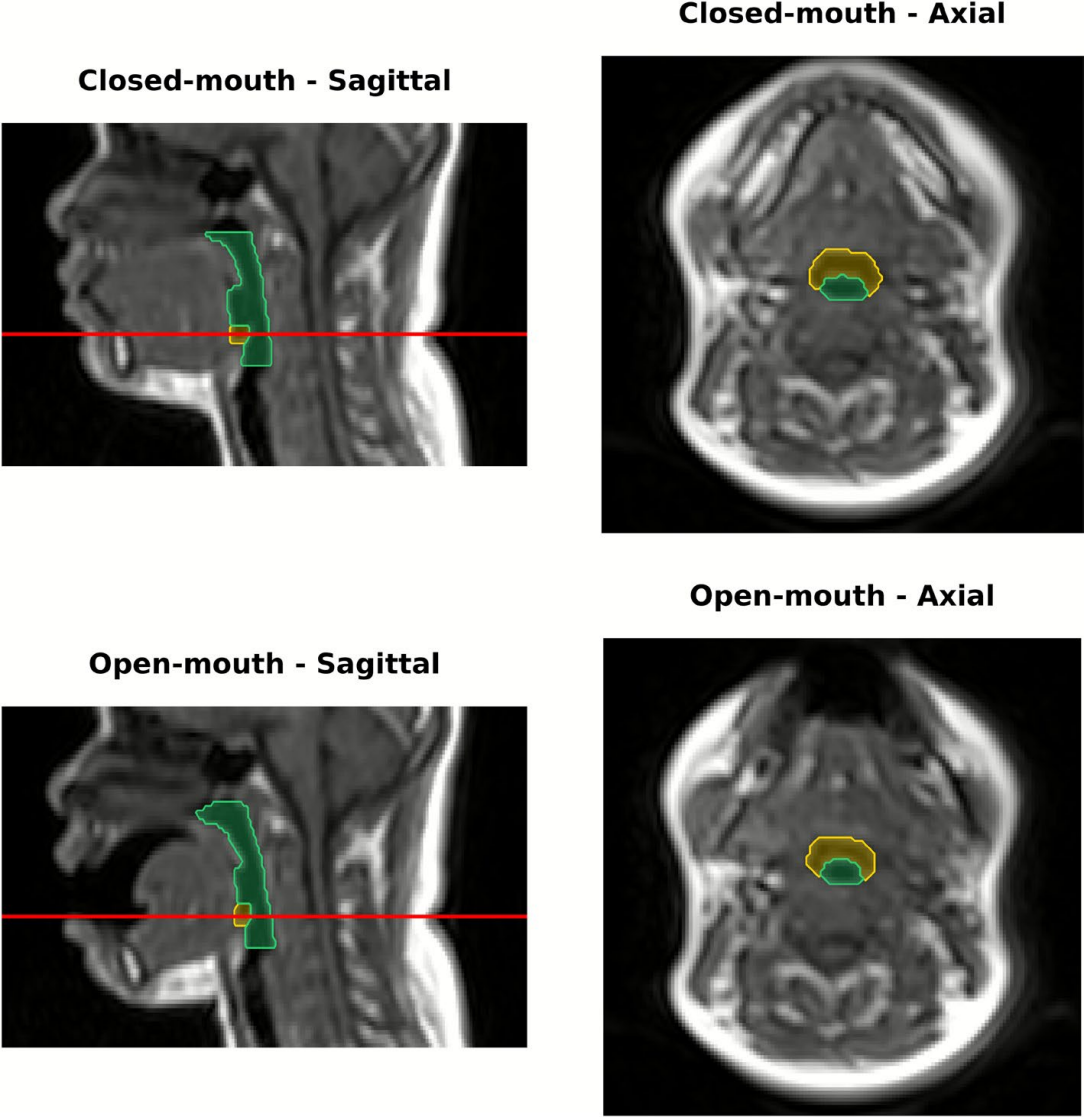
Representative sagittal (left) and axial (right) MR images of the upper airway in the closed (top) and open (bottom) mouth states with automated segmentations from the fourth‐generation model. The airway from 1 cm above the hard palate to 1 cm below the epiglottic base is overlaid in yellow, while the epiglottic airway alone is highlighted in green. On sagittal images, the red horizontal line indicates the axial slice level shown in the corresponding panels.
